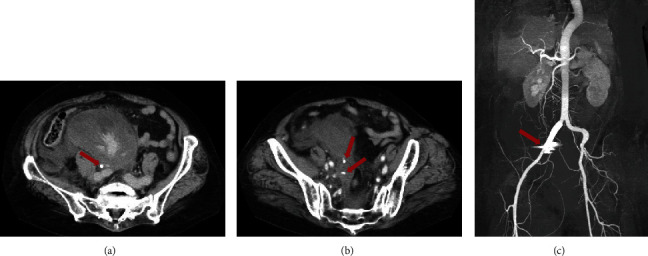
Contrast-enhanced CT reveals a large retroperitoneal hematoma and several bleeding points in the pelvis. (a) Bleeding point in retroperitoneal hematoma. (b) Bleeding points in retroperitoneal hematoma. (c) Bleeding surrounding the right iliac artery. CT, computed tomography.
